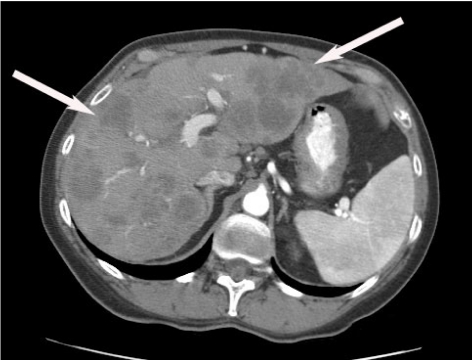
Pre-Y90 microsphere therapy planning evaluation in a 58 year old female with hepatic metastatic colorectal cancer. Contrast enhanced axial CT scan demonstrates numerous bilobar low attenuation lesions consistent with metastases. Note patency of the portal vein.
